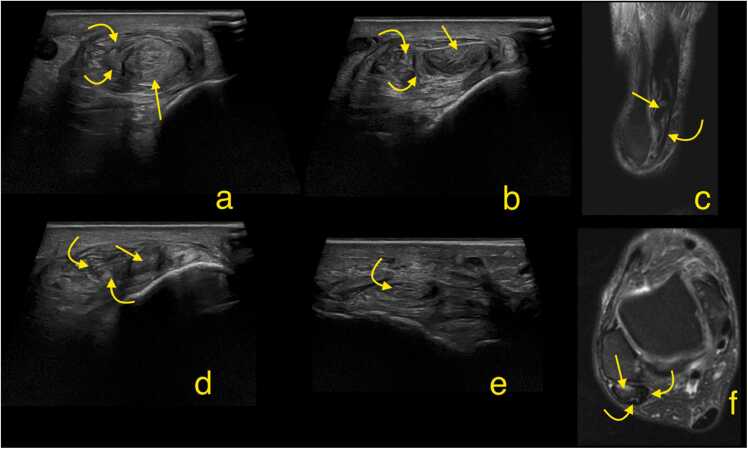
A patient with a history of an ankle sprain about one year ago, presenting with pain and limited range of motion in the ankle. Ultrasound (a, b, d, e) and magnetic resonance imaging (c and f) revealed a rupture of the peroneus brevis tendon with retraction of the tendon above the lateral malleolus (straight arrows). The peroneus longus tendon exhibits a split tear with small fragments (curved arrows) surrounding the stump of the peroneus brevis. Ultrasound transverse sections at the level of lateral malleolus (a and b). Magnetic resonance imaging: c- sagittal section, proton density-weighted with fat suppression showing fibula and peroneal tendons, d- slightly oblique section near the apex of the lateral malleolus, e- oblique section parallel and perpendicular to the peroneus longus tendon, f- transverse section, proton density-weighted with fat suppression at the level of lateral malleolus.
